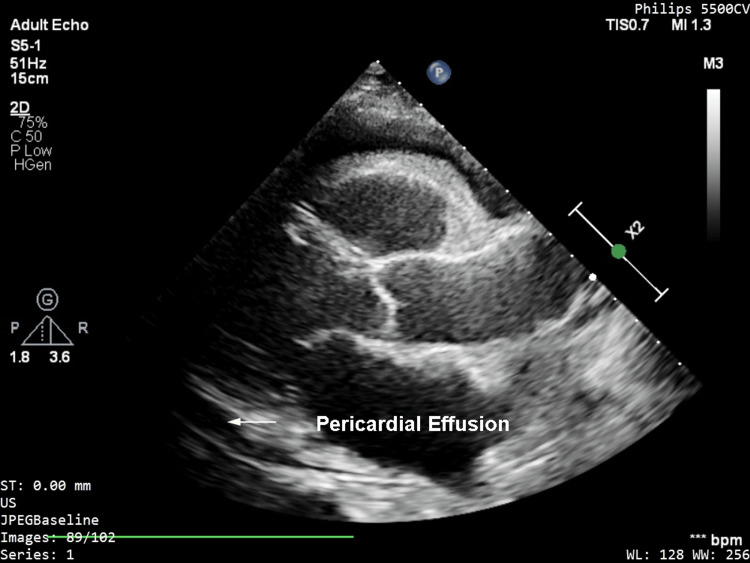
Transthoracic echocardiography showing moderate-size pericardial effusion
